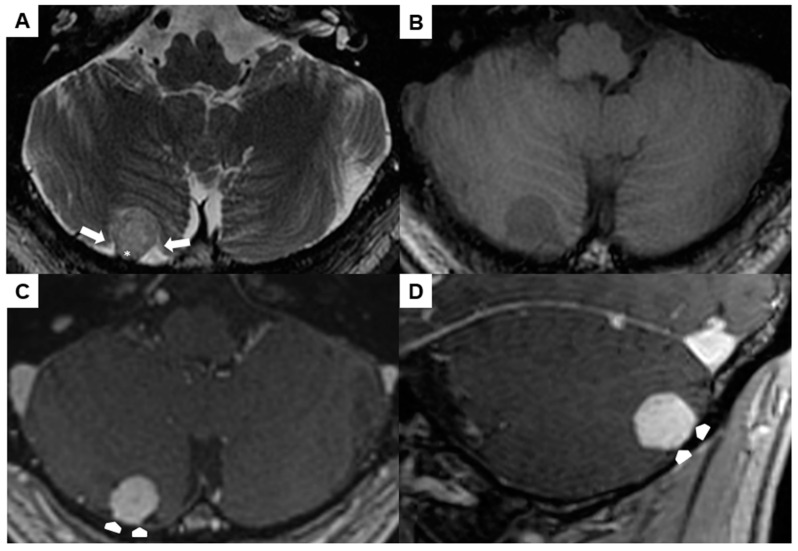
Preoperative magnetic resonance imaging of Case 2: The venous hemangioma showed a slightly hyperintense signal in the T2-weighted sequence (A), hypointense signal in the unenhanced T1-weighted sequence (B), and distinct contrast enhancement (C,D). Perifocal cerebrospinal fluid (arrows in (A)) and broad dural contact (asterisk in (A), arrowheads in (C,D)) proved the extra-axial location.
